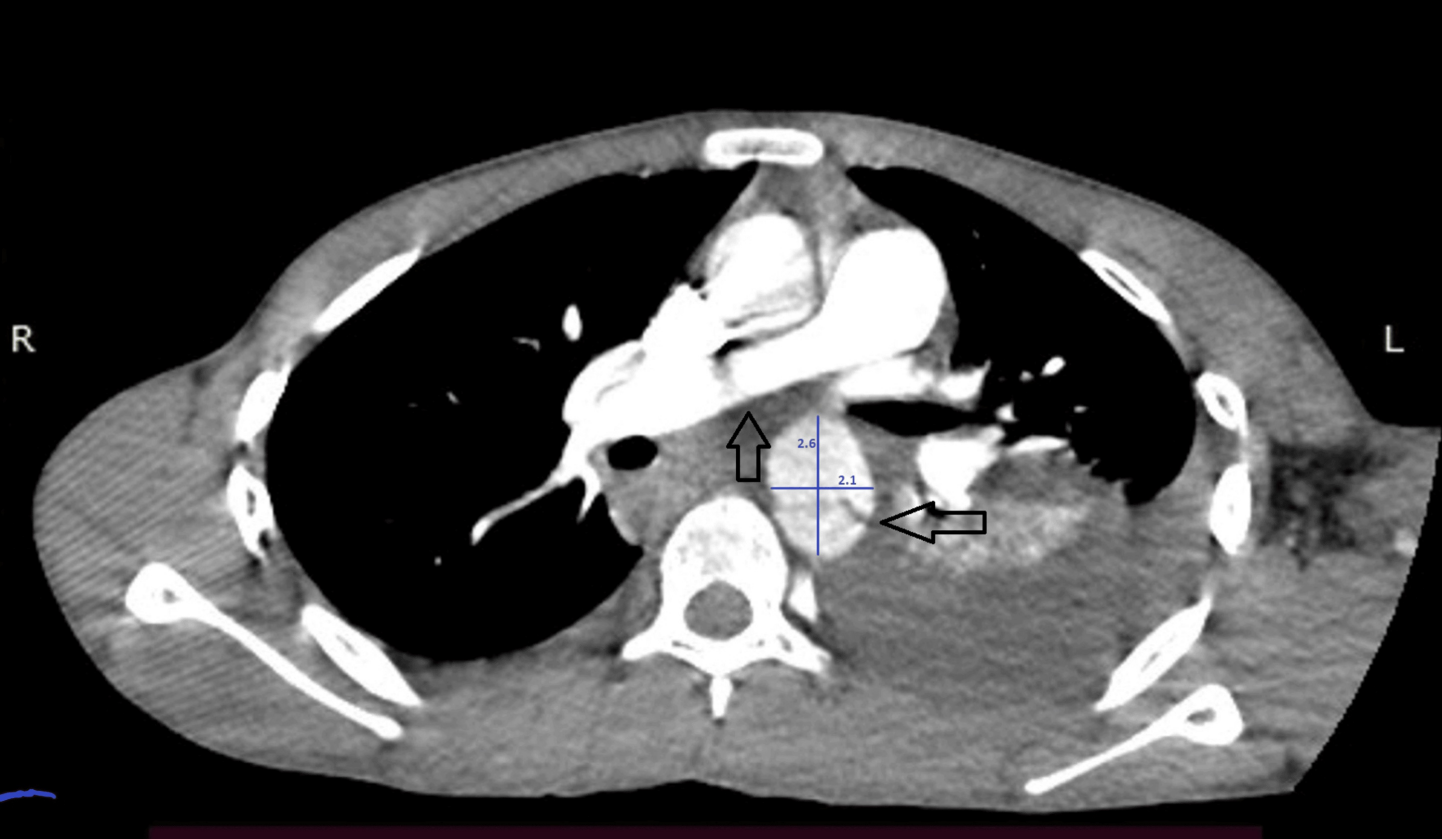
Preoperative computed tomography angiography (CTA) imaging Axial contrast-enhanced CTA images showing an intimal flap within the proximal descending thoracic aorta distal to the origin of the left subclavian artery, creating true and false lumens consistent with a traumatic Stanford type B aortic dissection (arrows). An associated left pleural hemothorax is also visualized.
